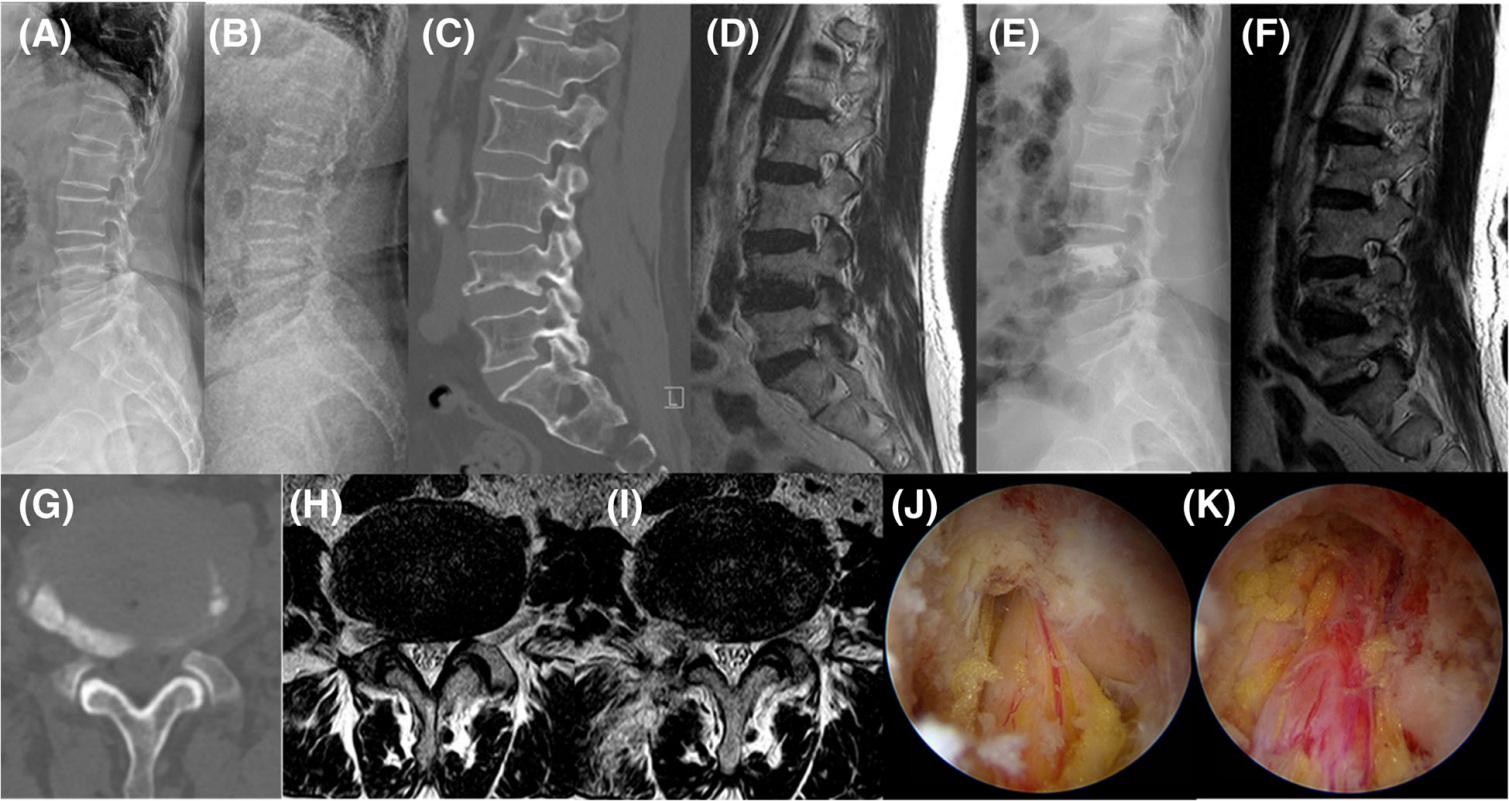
Acute L2 vertebral compression fracture sustained 2 months after surgery and treated with percutaneous VP. An 81-year-old female patient was diagnosed with L4 osteoporotic vertebral collapse (flat-type) and severe central canal and right central hugged lumbar disk herniation at L4-L5 underlying lumbar degenerative kyphosis. (A) Whole-spine lateral radiographic image (white dotted line: plum line). The patient simultaneously underwent (B) L4 percutaneous balloon kyphoplasty and (C) unilateral biportal endoscopic decompressive laminectomy. Sufficient neural decompression is seen on the magnetic resonance T2-weighted axial images (D) preoperatively, (E) immediately postoperatively, and (F) after 2 months postoperatively. Within the 2 months after surgery, she felt a significant improvement in pain and disability. Plain lateral radiographs (G) immediately postoperatively and (H) at 2 months postoperatively show acute L2 vertebral compression fracture, and (I) percutaneous vertebroplasty was performed on L2
